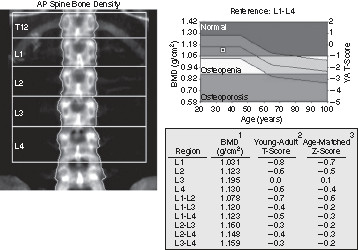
Dual Energy X-ray Absorptiometry (DXA) for Osteoporosis. This DXA display printout shows the results of a typical normal study. DXA scans are used to quantify bone mass that is expressed in standard deviations from the normal. This information can be applied to management, prognosis, and estimation of fracture risk in patients with osteopenia or osteoporosis. DXA is the most common study used as a screening test in women over age 65 and others at risk for osteoporosis. (Reprinted with permission from Taylor JAM, et al, Skeletal Imaging, Atlas of the Spine and Extremities. 2010, 2^nd^ edition. St. Louis, Elsevier.).
